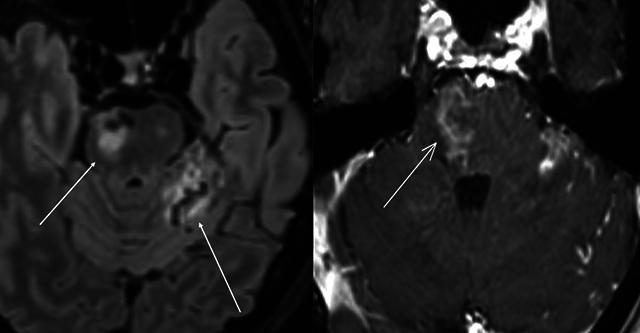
FLAIR and post-contrast T1 weighted images showing a hemorrhagic lesion in the right anterior pontine region, with surrounding edema and contrast enhancement, indicative of venous infarction.
